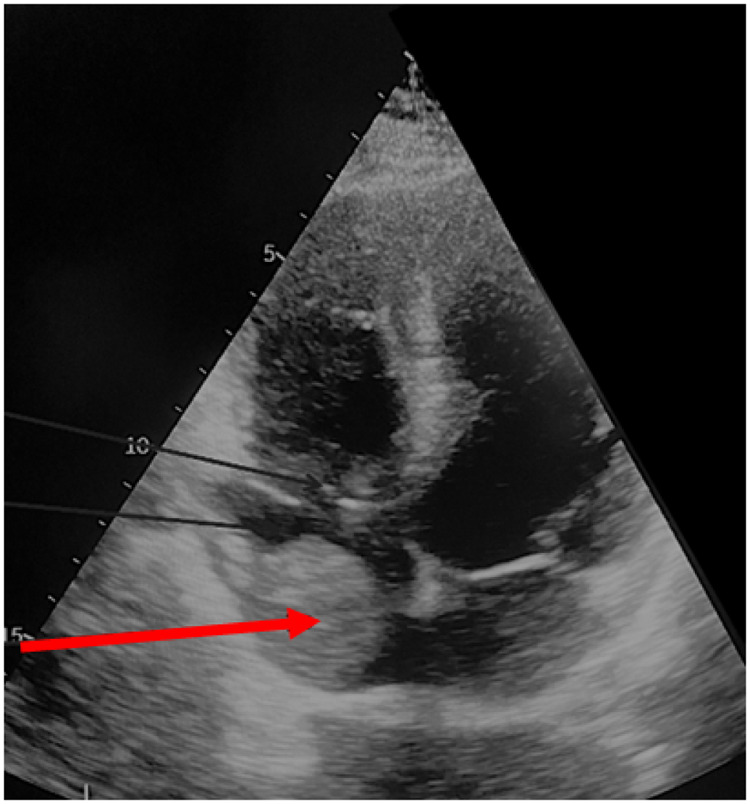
Echocardiogram showing a tumor thrombus in the right atrium (red arrow).
